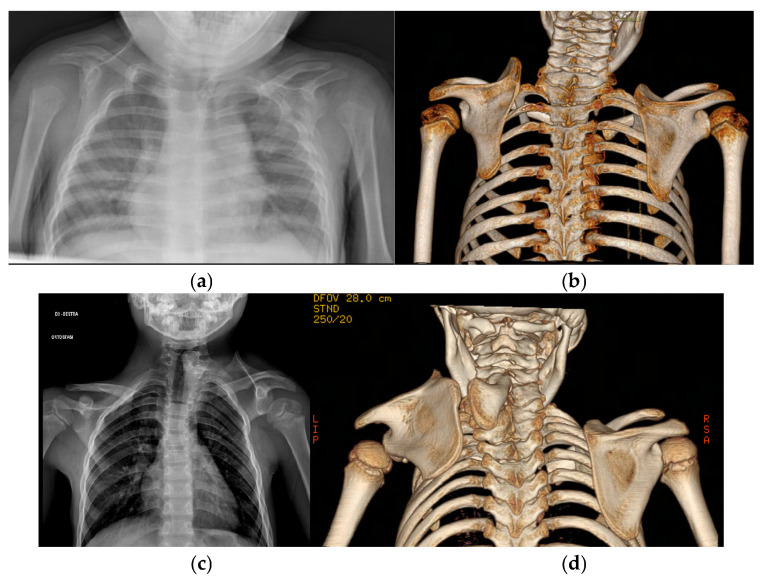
Radiographic imaging of Sprengel’s shoulder: (a) plain AP radiograph shows Type 2 SD according to Rigault with scapula apex at the C5 level; (b) the 3D CT scan shows elevation of the left scapula, with no vertebral connections; (c) plain AP radiograph shows Type 3 SD according to Rigault with scapula apex at the C4 level; (d) the 3D CT scan reveals the presence of an omovertebral bone and multiple cervical fusions on the right side, consistent with Klippel–Feil syndrome.
